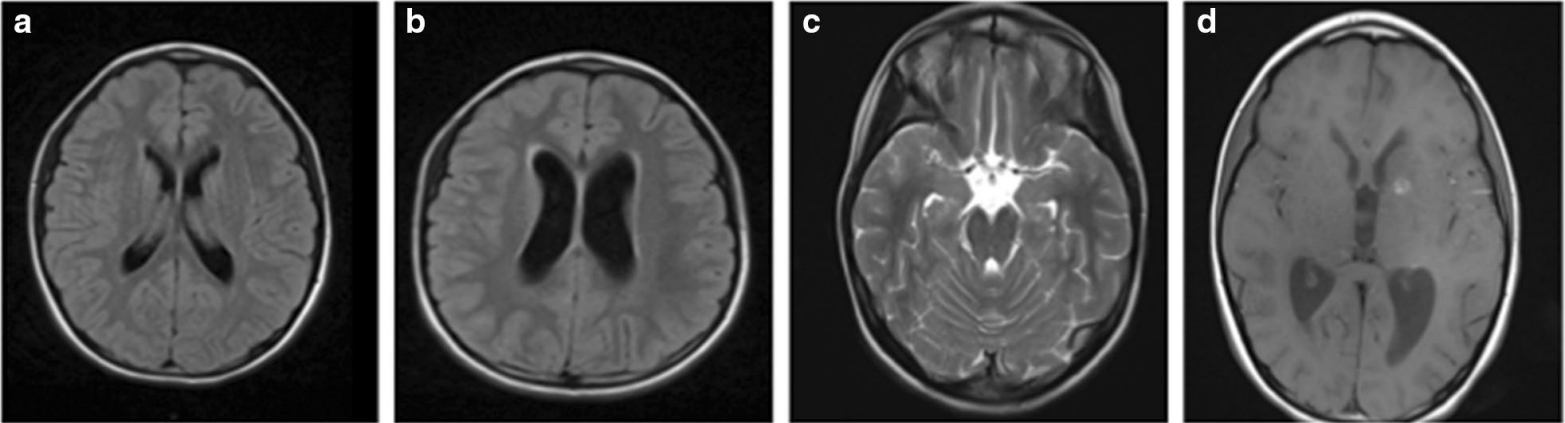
Brain magnetic resonance imaging (MRI). At the onset of the symptoms normal imaging was found (a), after 2 days leptomeningeal enhancement over the basilar cistern and hydrocephalus were relieved (b, c). At admission day 27, focal areas of signal restriction in correspondence of the left caudate nucleus and of the posterior arm of the left internal capsule (d) were found suggesting a tuberculous cerebral vasculitis (TVC) as a complication
